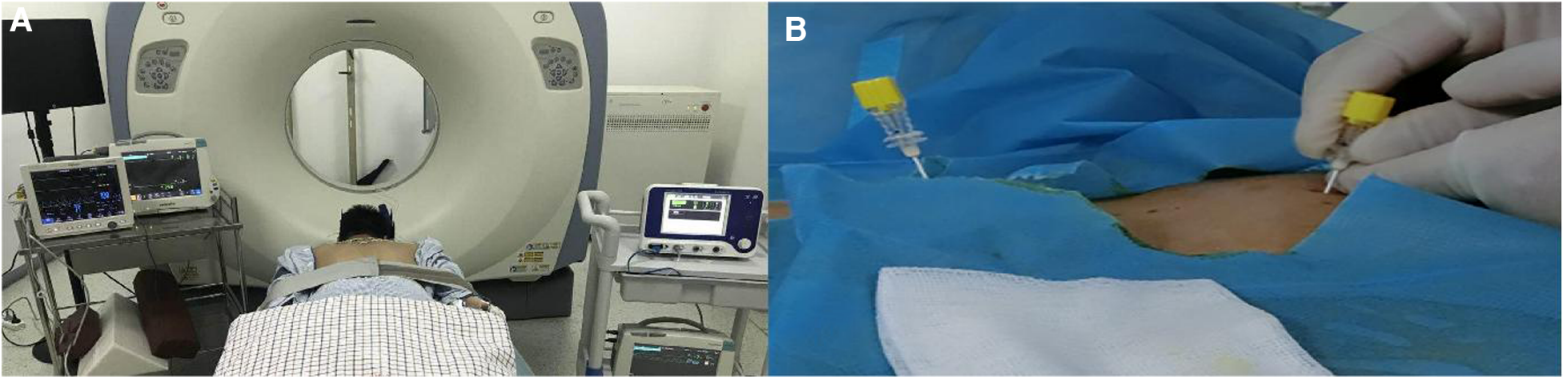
(A): Patient in prone position under CT guidance; (B): The puncture needle is inserted from the chest and back.
